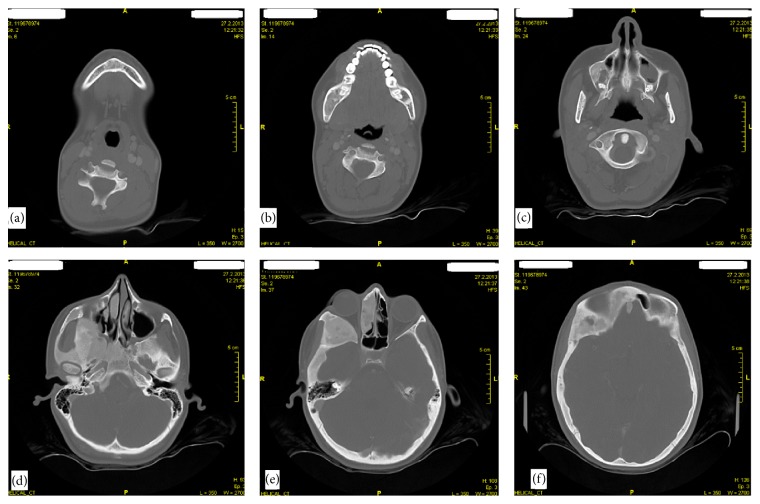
(a), (b), (c), (d), (e), (f) Axial CT scans (bone window) showing multiple bone lesions involving skull base and facial bones.
